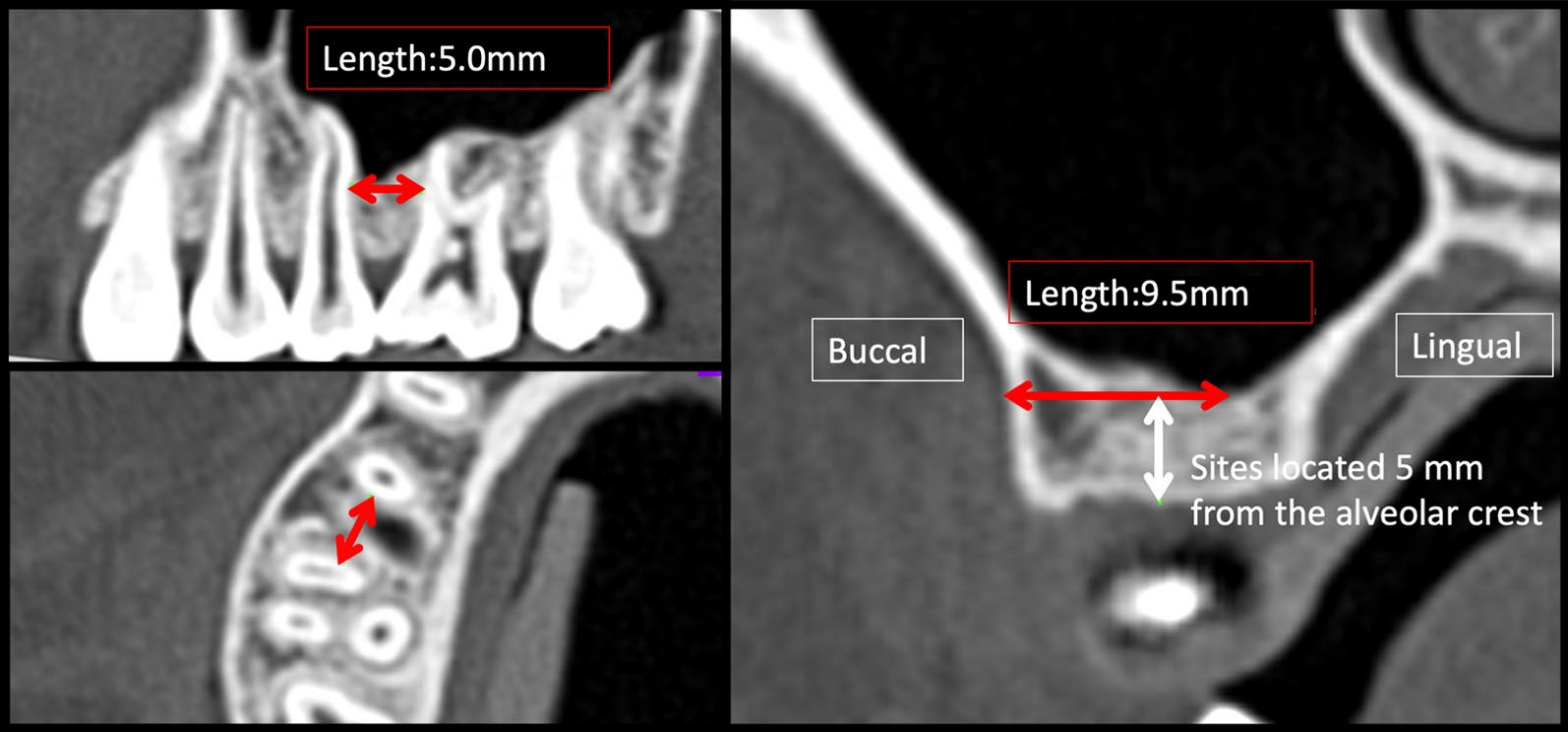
Measurements on CT images. Maxillary buccal alveolar bone thickness and distance between the roots of the maxillary second premolar and first molar at 5, 6.5, and 8 mm from the alveolar crest were measured on CT images
